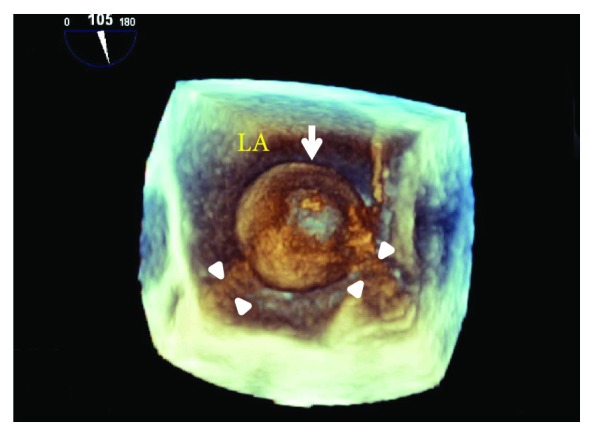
Three-dimensional transesophageal echocardiographic image of the free-floating ball-shaped mass (arrow) around which some sludge (arrow heads) is present suggesting a potential mechanism of its formation. Abbreviations are the same as in Figure 2.
